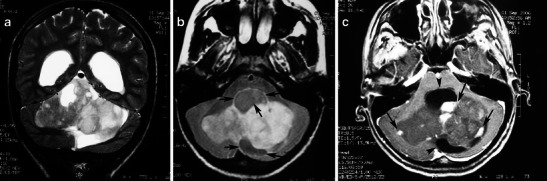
A pilocytic astrocytoma with a predominantly solid mass and a minimal cyst-like component in a 14-year-old girl. a This coronal T2-weighted image shows a large, well-marginated mass, involving the vermis and both cerebellar hemispheres that effaces the fourth ventricle. b On an axial FLAIR image, the solid mass shows high signal intensity with hypointense cystic areas (arrows). c On an axial contrast-enhanced image, the mass shows inhomogeneous contrast enhancement, with nodules that exhibit intense enhancement (arrows) and other cystic areas that remain unenhanced (arrowheads)
